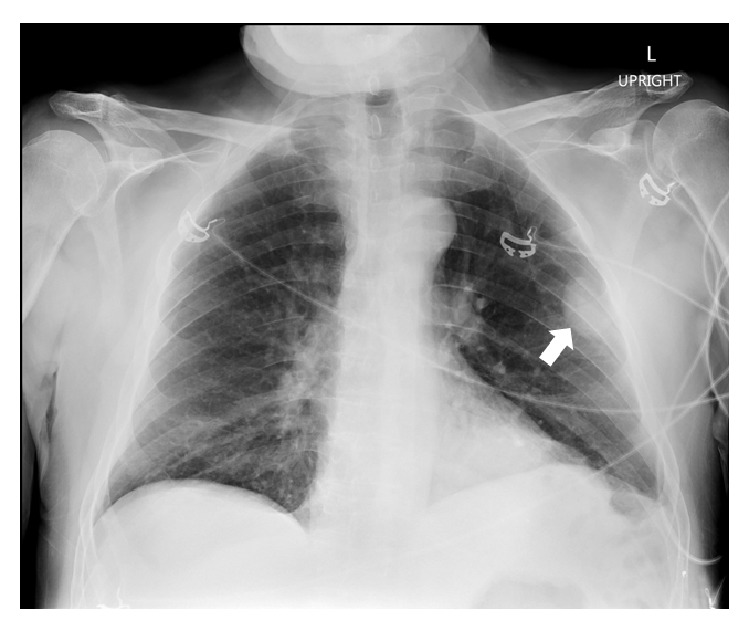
Anteroposterior Chest X-ray showing left sided pleural-based mass.
